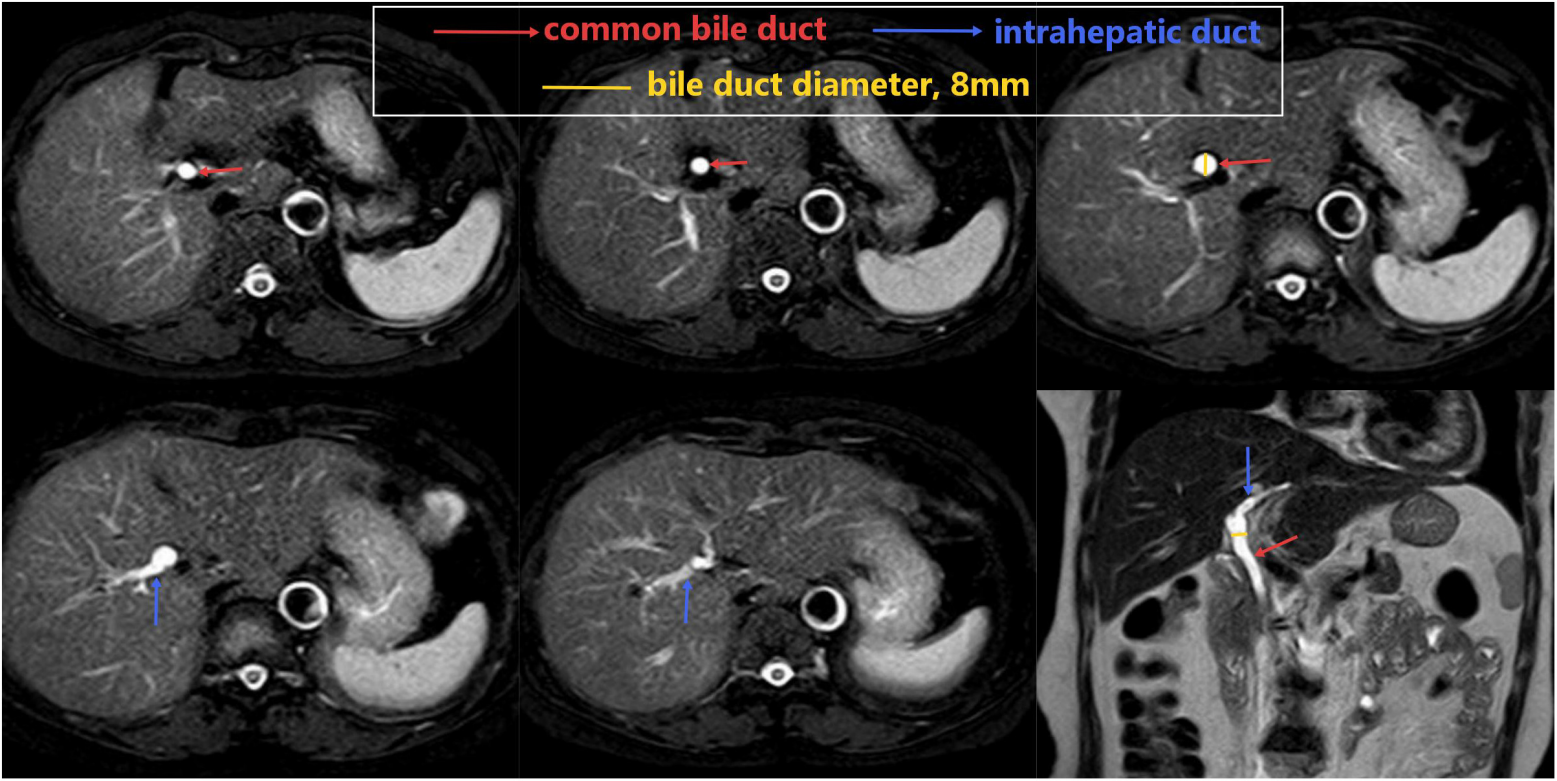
Magnetic resonance imaging (MRI) showed no clear gallbladder structure is visible, and the bile duct diameter is at the upper limit. From the lower end of the bile duct to the intrahepatic bile duct, the continuity of the bile duct wall is visible, and no cystic duct branches are seen.
